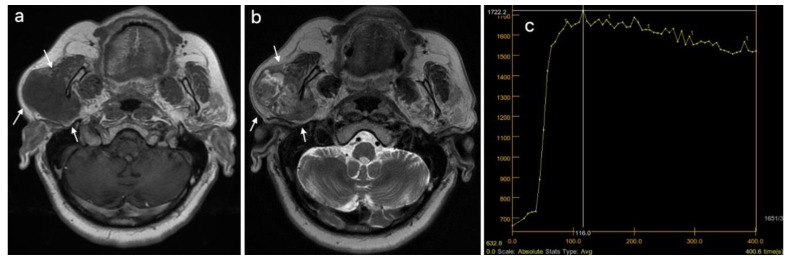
Malignant tumor of the right parotid gland (white arrows): (a) T1WI sequence showing an inhomogeneous hypointense signal in the tumor, with infiltrative borders; (b) T2WI sequence also showing an inhomogeneous signal, with central necrotic areas; (c) DCE-MRI showing a type C TIC curve.
